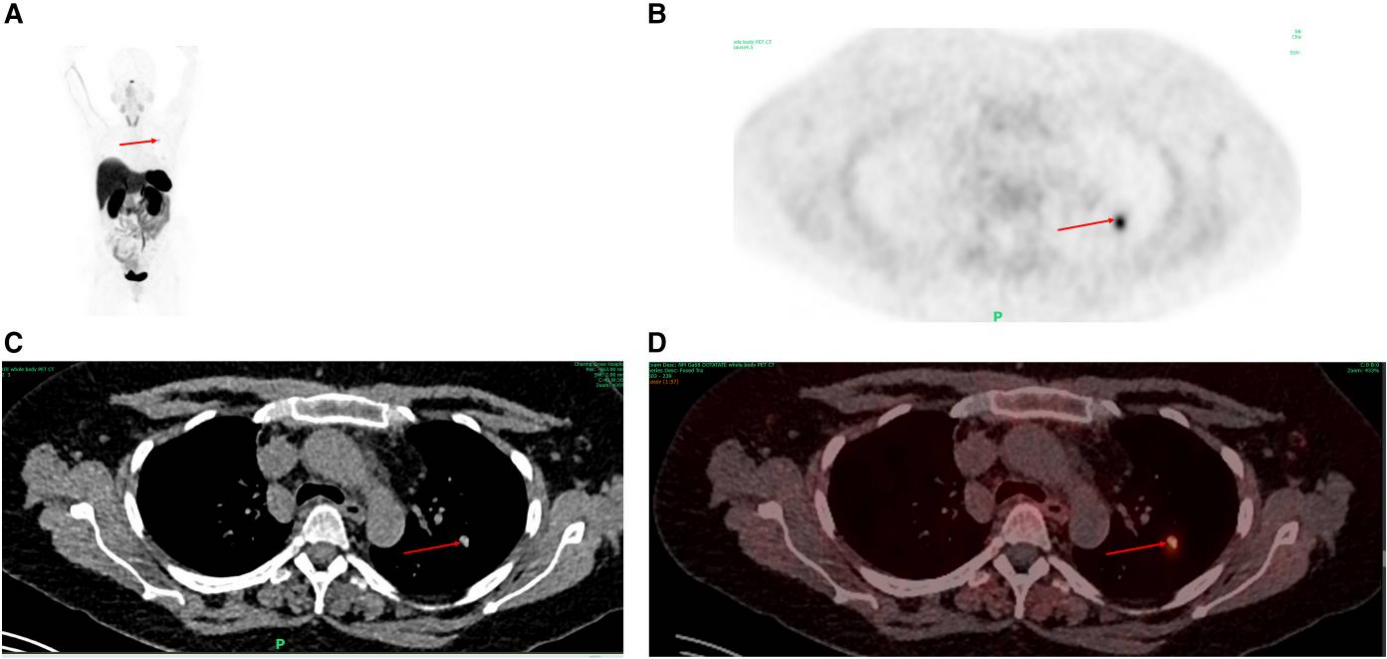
A, Maximum intensity projection of gallium68-DOTATATE scan; B, axial positron emission tomography; C, axial computed tomography; and D, fused image, with arrows pointing at the culprit lesion in all views.
